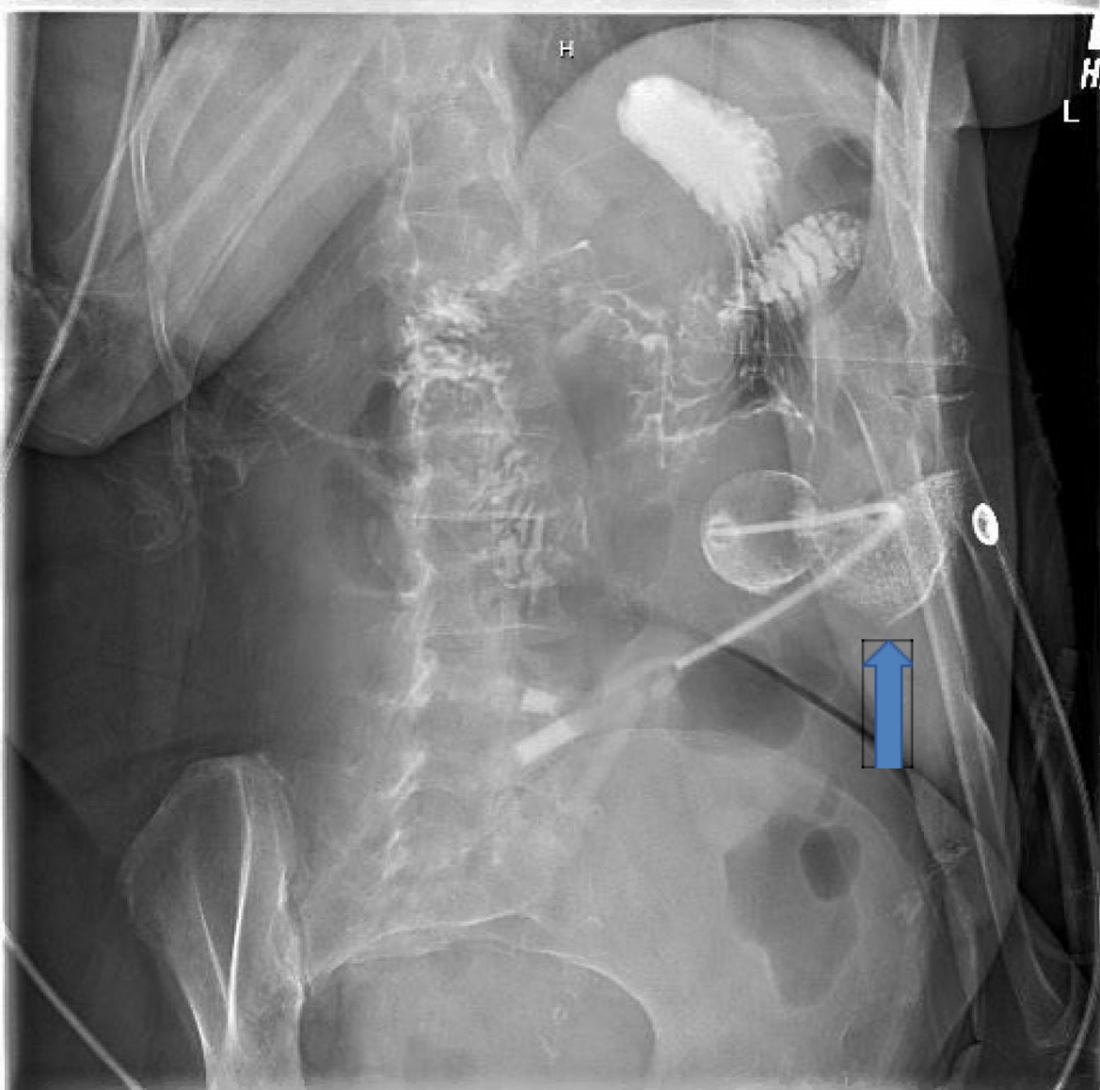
Gastrostomy X-ray With Gastrografin Injection Through PEG Tube Showing Extravasation at the Skin Surface (Blue Arrow)
